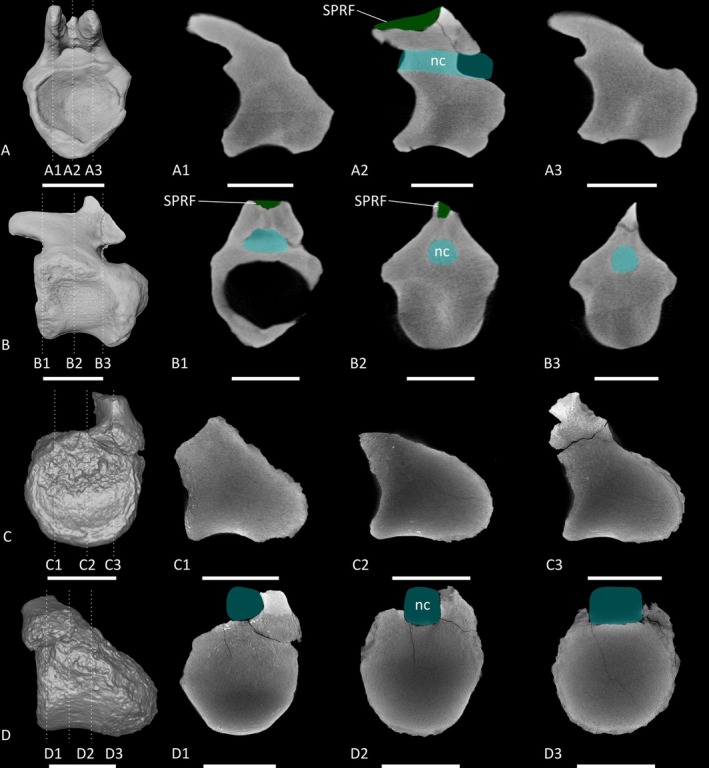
CT scan of indeterminate titanosaur caudal vertebrae. (A, B) 3D rendering, (C, D) surface scan; (A, C) anterior view, (B, D) left lateral view; (A, B) Titanosauria gen. et sp. indet. anterior caudal vertebra MSNM V7157, (C, D) Eutitanosauria gen. et. sp. indet. anterior caudal vertebra BAS D.8621.25; (A1–A3, C1–C3) CT scan slices in parasagittal cross‐section, (B1–B3, D1–D3) CT scan slices in transverse cross‐section. Nc, neural canal; SPRF, spinoprezygapophyseal fossa. Key: Blue highlight, neural canal; green highlight, neural spine fossa. Scale bars = 50 mm.
